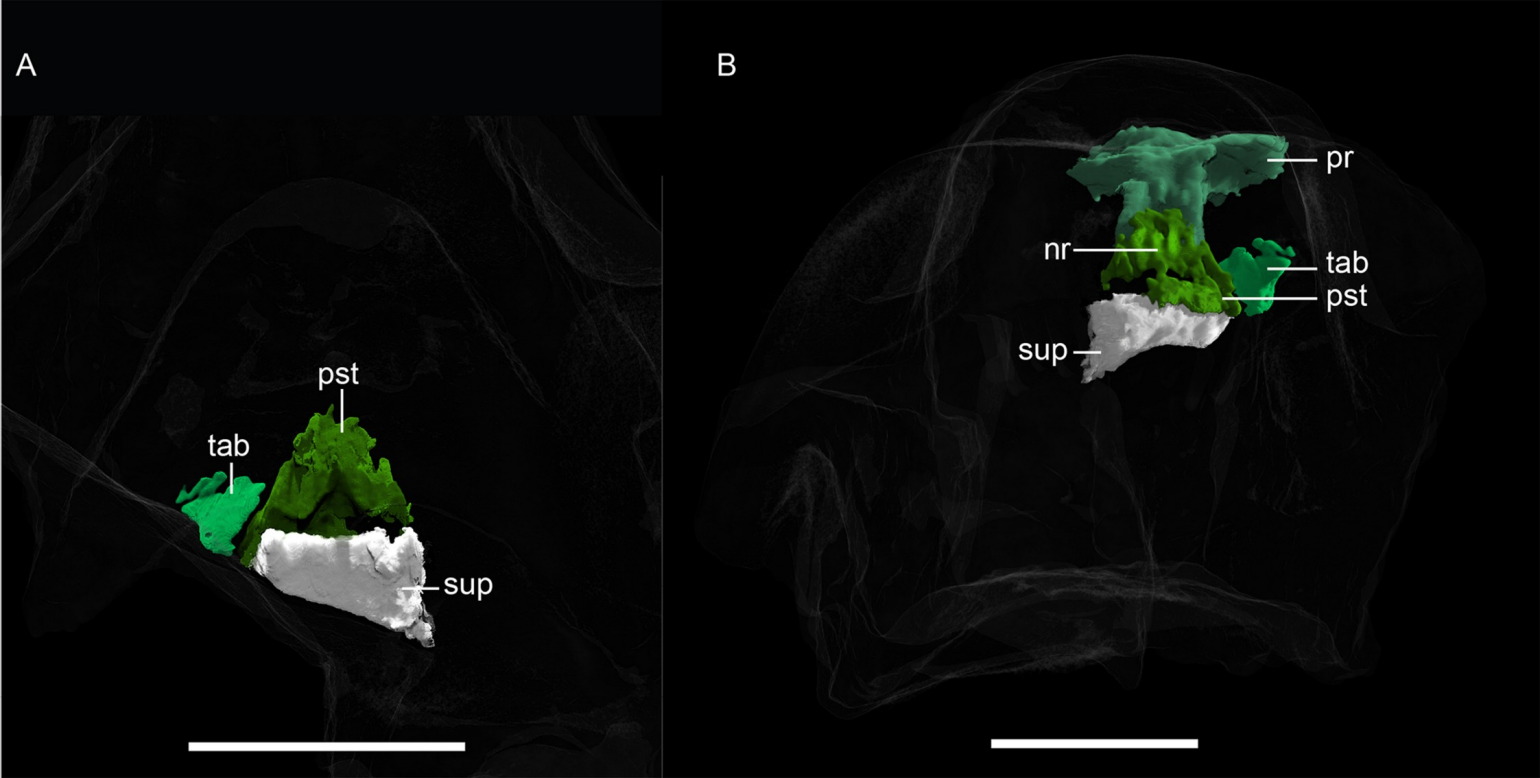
Neutron CT reconstruction of MB.R.999: rear end of skull roof from (A) ventral and (B) dorsal. Abbreviations: nr, nuchal ridge; pr, parietal; pst, postparietal; sup, supraoccipital; tab, tabular. Scale bars = 2 cm.
